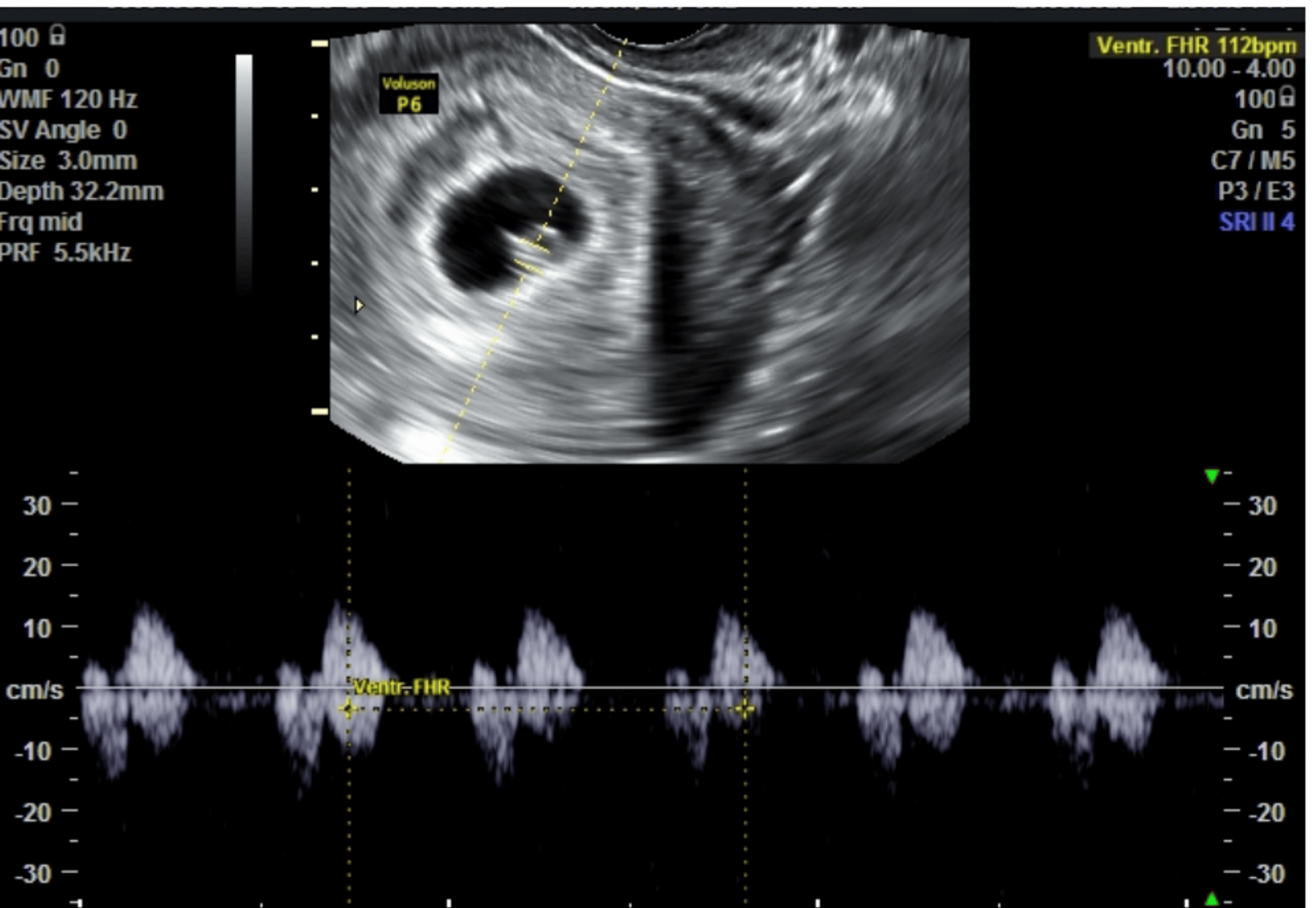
Transvaginal ultrasound (Case 1, 6.5 weeks) demonstrating a 2.3 × 1.7 cm subchorionic hematoma adjacent to a live intrauterine gestation.
